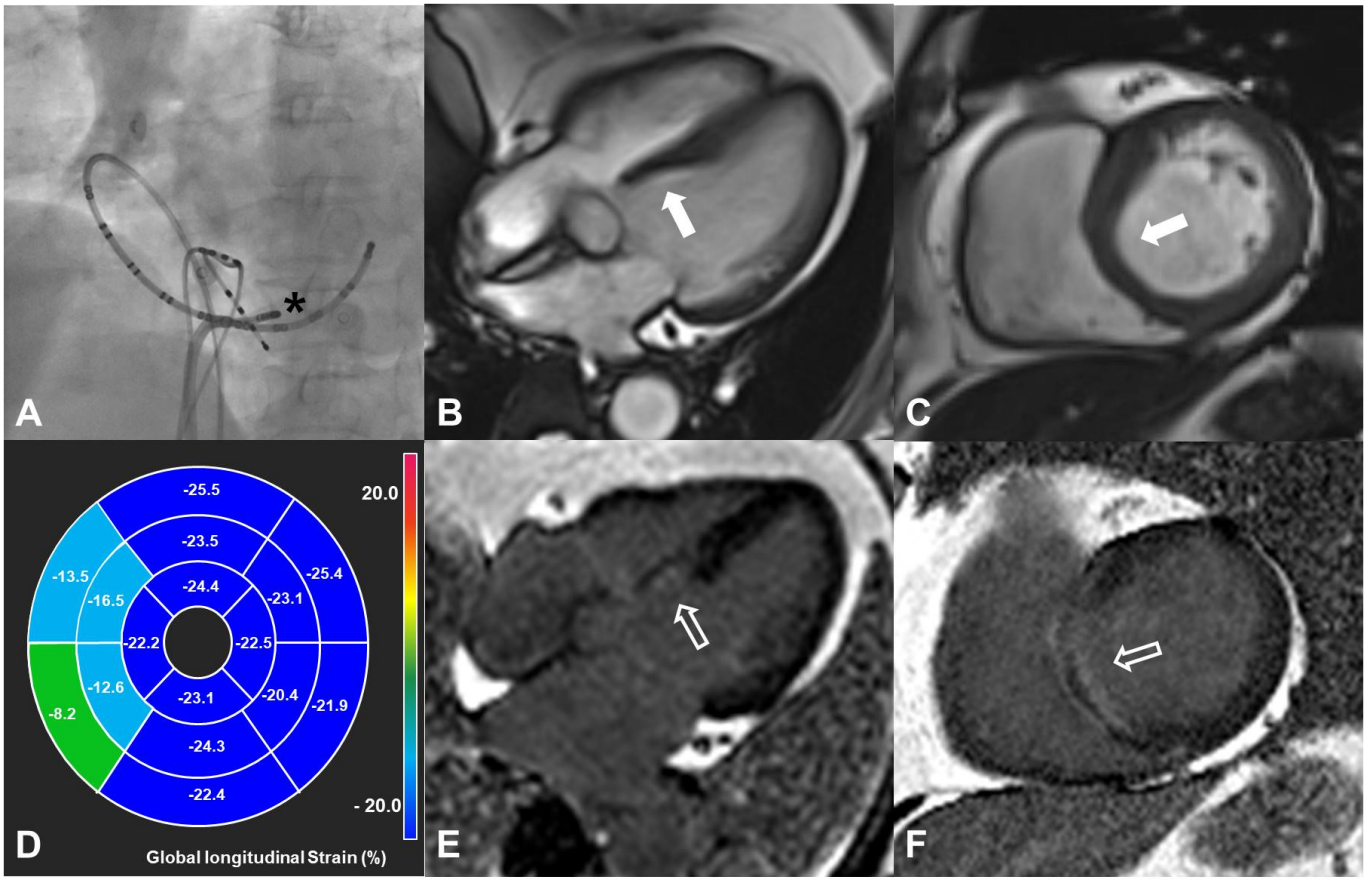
A 67-year-old female patient with Wolff-Parkinson-White syndrome. She underwent an electrophysiologic study due to frequent palpitations. Radiofrequency ablation was applied to the posteroseptal accessory pathway through the transseptal approach (asterisk) (A). One year later, the patient underwent cardiac magnetic resonance imaging. Four-chamber and short-axis cine images show regional myocardial wall thinning (about 5.1mm) at the basal inferior septum on the end-diastolic phase (arrows) (B, C). Decreased global longitudinal strain is noted at the basal septum with the feature tracking method, which suggests regional akinesia (D). The left ventricular ejection fraction is 47.6%. Linear endocardial and epicardial late gadolinium enhancements not corresponding with the vascular territory are noted on the four-chamber and short-axis late gadolinium enhancement images (open arrows) (E, F).
